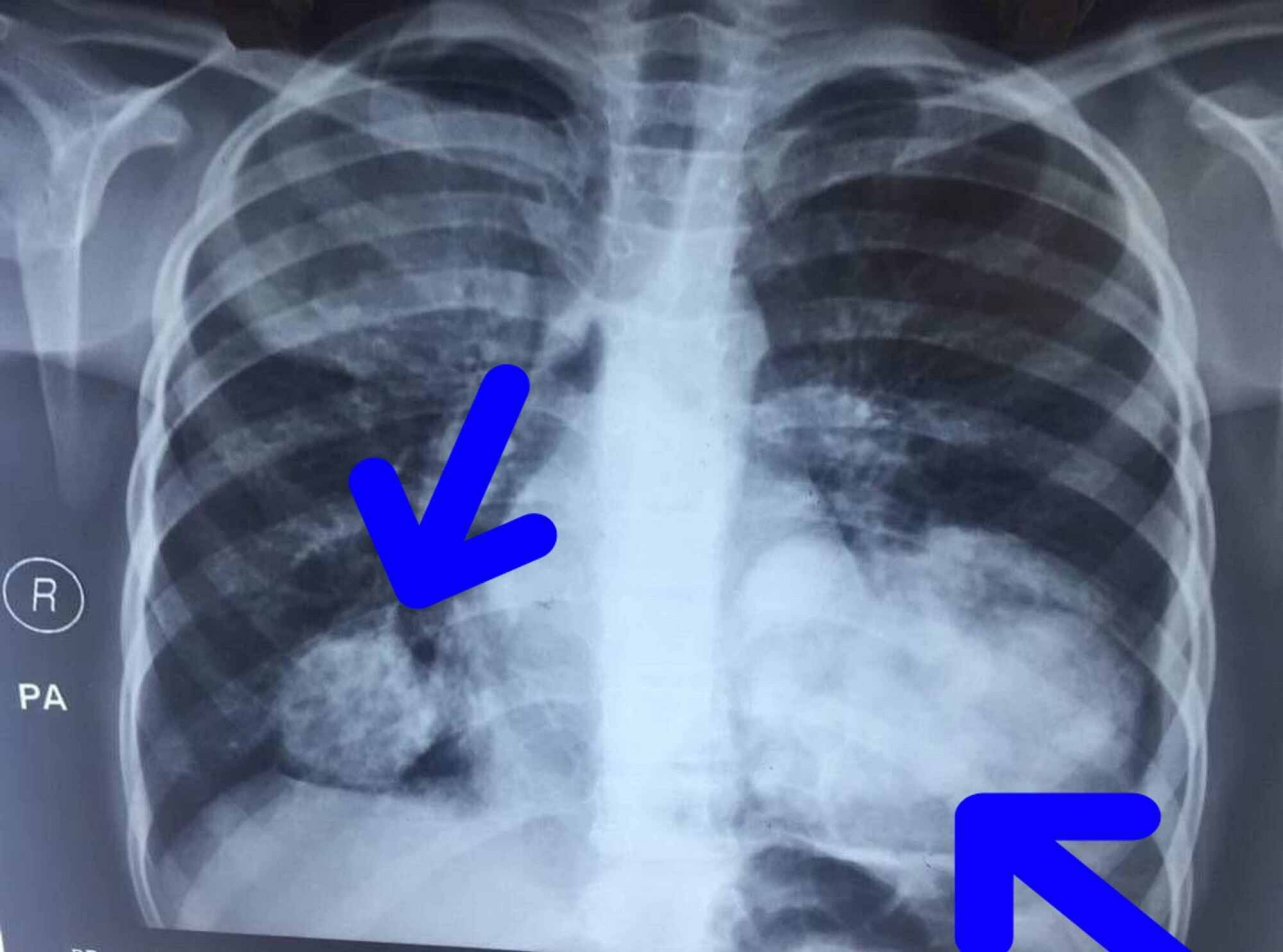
A chest X-ray revealed bilateral multiple dense masses of varying sizes and a small left-sided pleural effusion suggestive of metastasis
